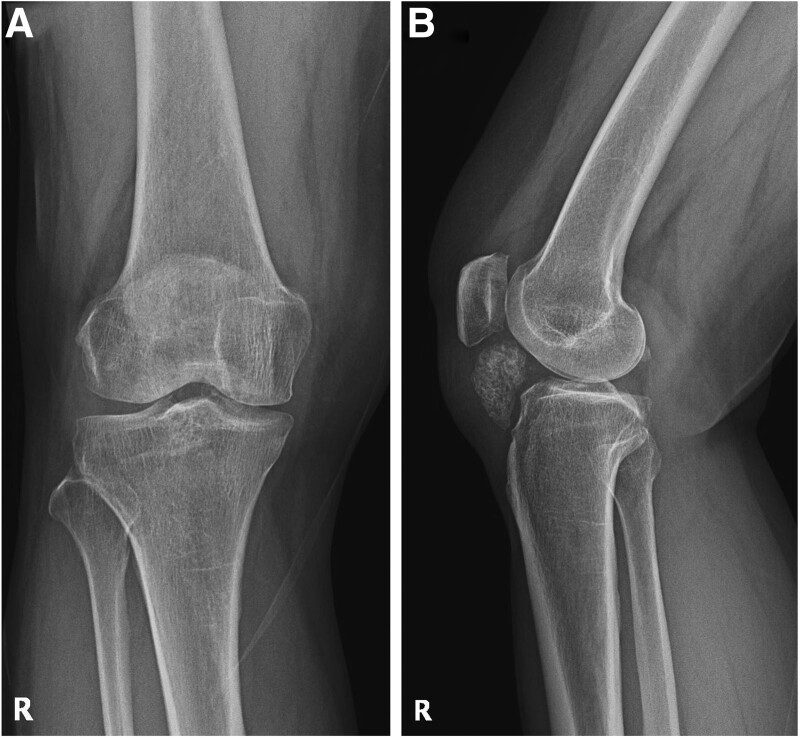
The simple radiography images show a mass with calcification in the infrapatellar fat pad of the right knee. AP view (A), Lateral view (B).
